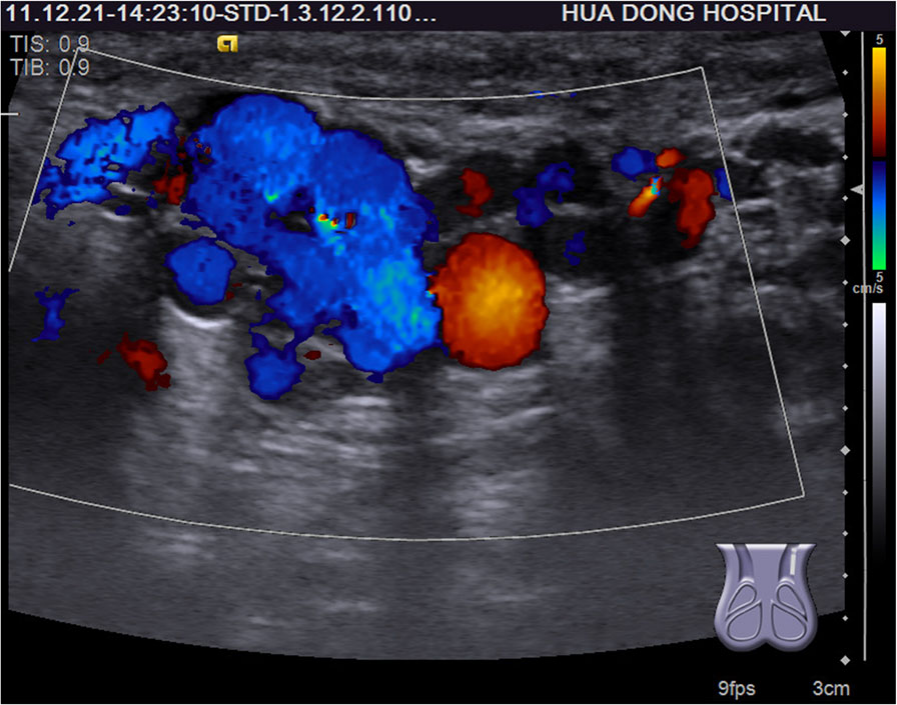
The varicocele showed in the ultrasonography
